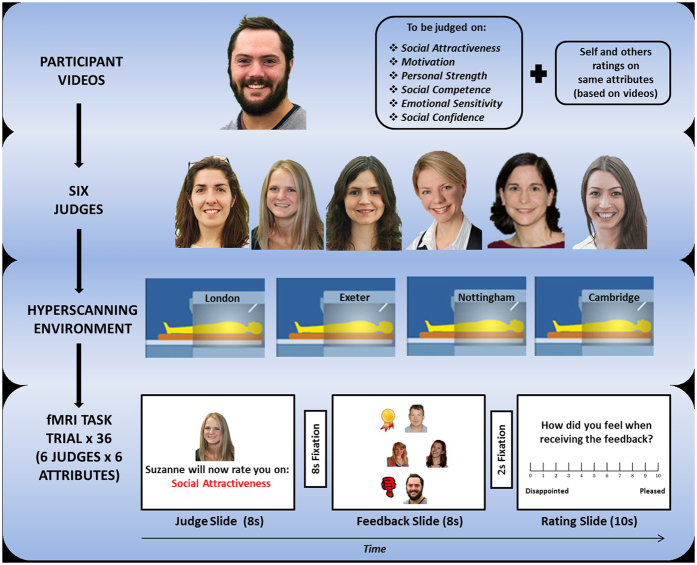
The Social Feedback Task. Participants (*n* = 56; Table S1) were first instructed to make 1-minute videos in which they talk freely about themselves and their aspirations. Participants believed their performance in these videos was being rated on six social attributes by a panel of six judges (confederates) in comparison to 3 other contestants (also confederates). Participants believed that they and the other contestants were in MRI scanners across the UK (in fact, only the participants were being scanned). They were able to view (and rate) the videos of the other contestants (confederates) prior to entering the scanner^13^. In the scanner, in order to provide signals of social inclusion and social exclusion, participants received feedback from each judge on each social attribute relative to the other contestants and were then asked to rate how the feedback made them feel. Feedback was either socially positive/inclusive (rated the best of the four contestants on that attribute by that judge and positioned at the top of the Feedback Slide with an adjacent gold medal), socially negative/excluding (rated the worst on that attribute and positioned at the bottom of the Slide with a thumbs-down graphic), or neutral (rated intermediate and positioned in the middle of the Slide). Participants received equal amounts of such positive, negative and neutral social feedback during the course of the task.
